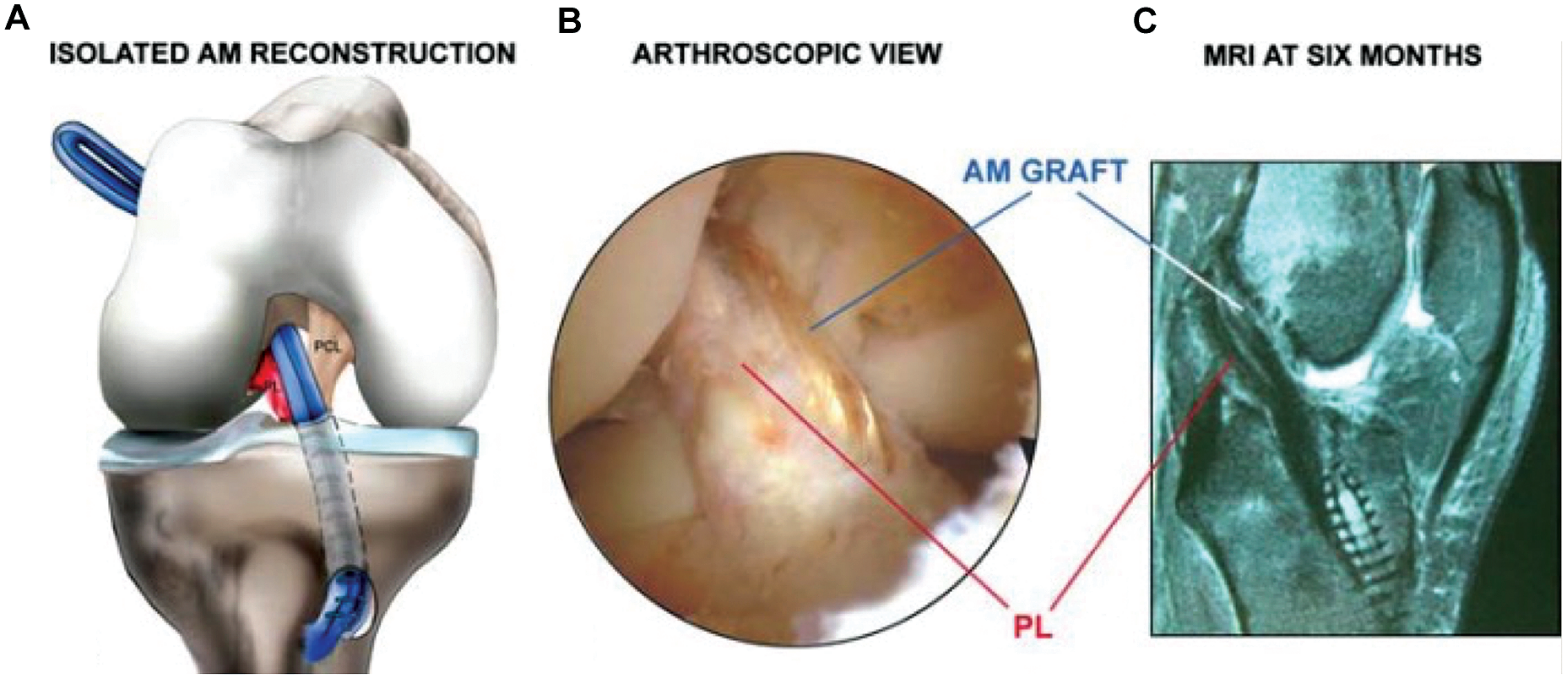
(A) The hamstring graft is routed from the tibia to the femur. The graft’s tibial insertion is preserved to improve further tibial fixation. (B) Arthroscopic view. (C) Magnetic resonance imaging (MRI) scan of the isolated anteromedial (AM) bundle reconstruction with the intact posterolateral bundle (PL) and the AM graft demonstrated.
